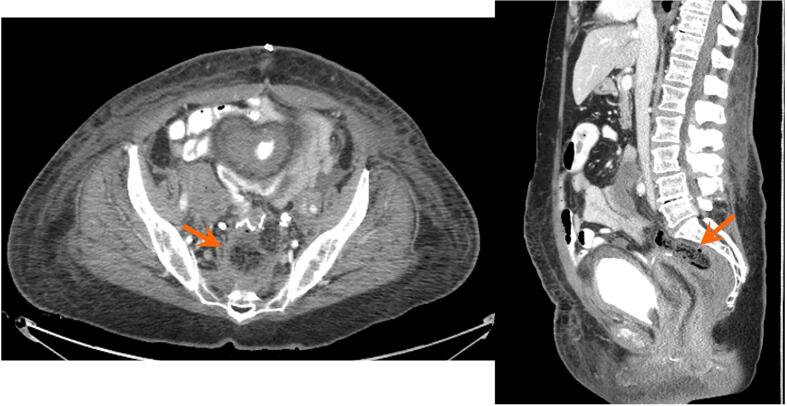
The first postoperative CT after colorectal anastomosis demonstrates a feculent-appearing collection posterior to the suture line (arrows), suspicious for anastomotic leak.
